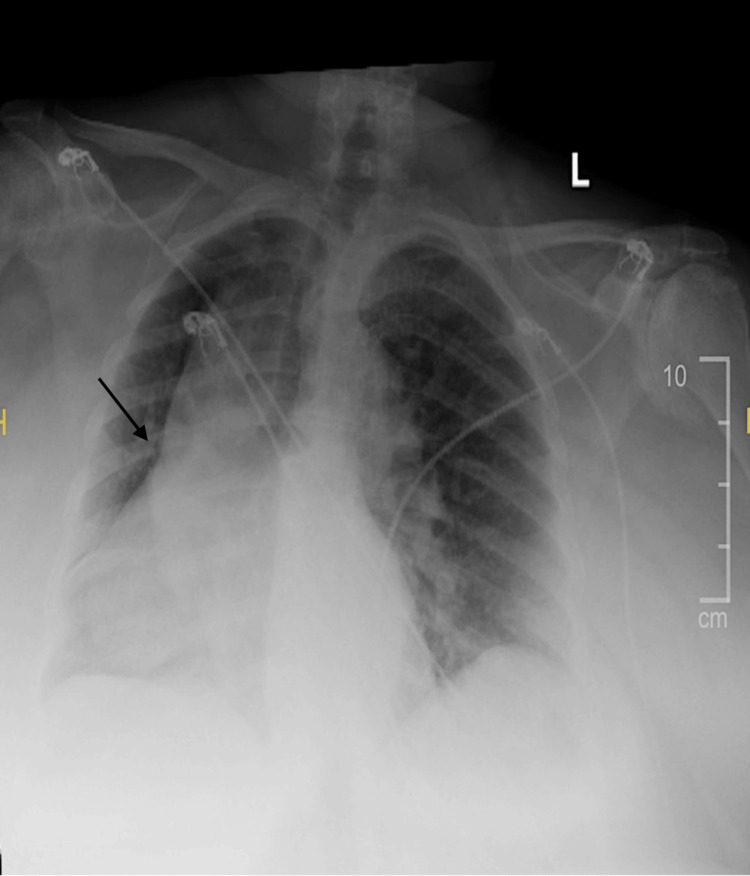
Chest radiograph demonstrating right middle lobe collapse with rightward displacement of the cardiac silhouette Black arrow indicates the region of right middle lobe atelectatic opacity/volume loss.
